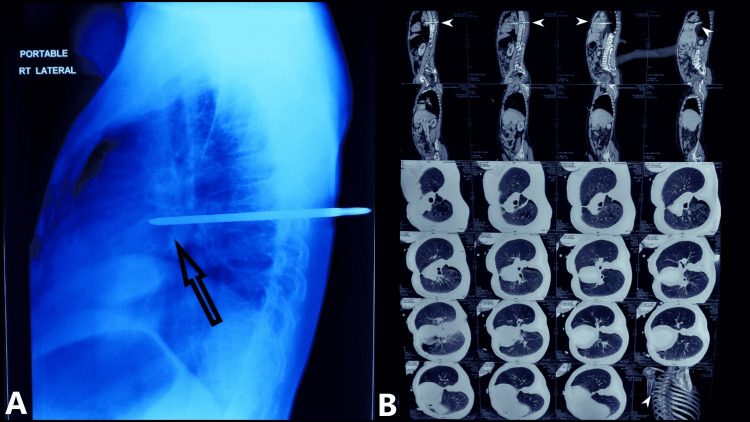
A: Chest X-ray showing the depth of penetration. B: Sagittal CT scan showing the screwdriver abutting the great vessels and transecting the spine as indicated by the white arrowheads.
